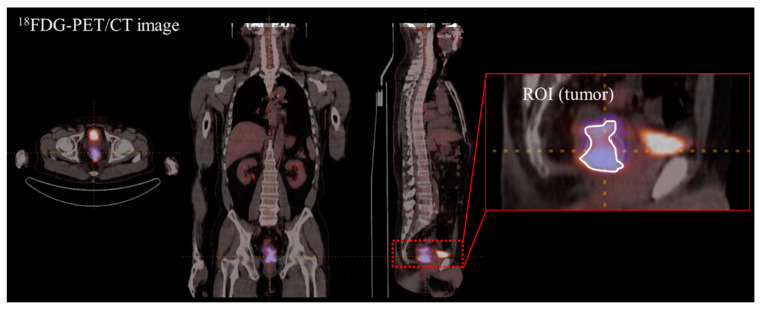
Radiomics extracted from ^18^F-FDG PET/CT.
